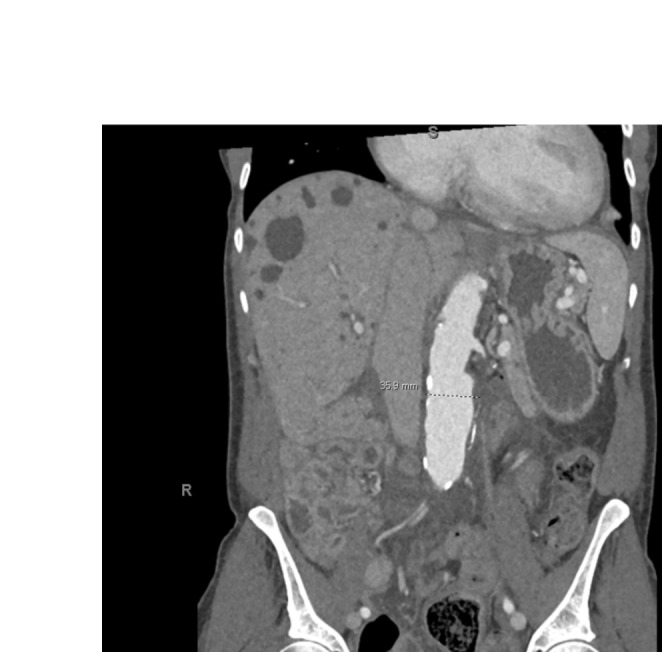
CT scan of the abdomen, coronal view showing postoperative changes and interval bilateral nephrectomy.
